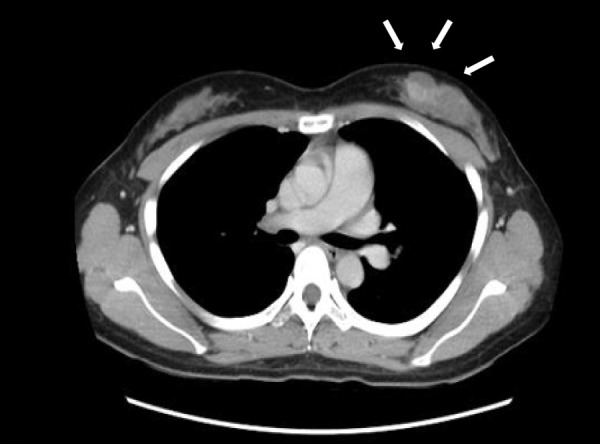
CT. CT examination showed a mass measuring 2 cm in diameter was present in the upper-inner quadrant of the left breast as indicated by the arrows. There were no findings suggestive of obvious lymph node enlargement or distant metastasis.
